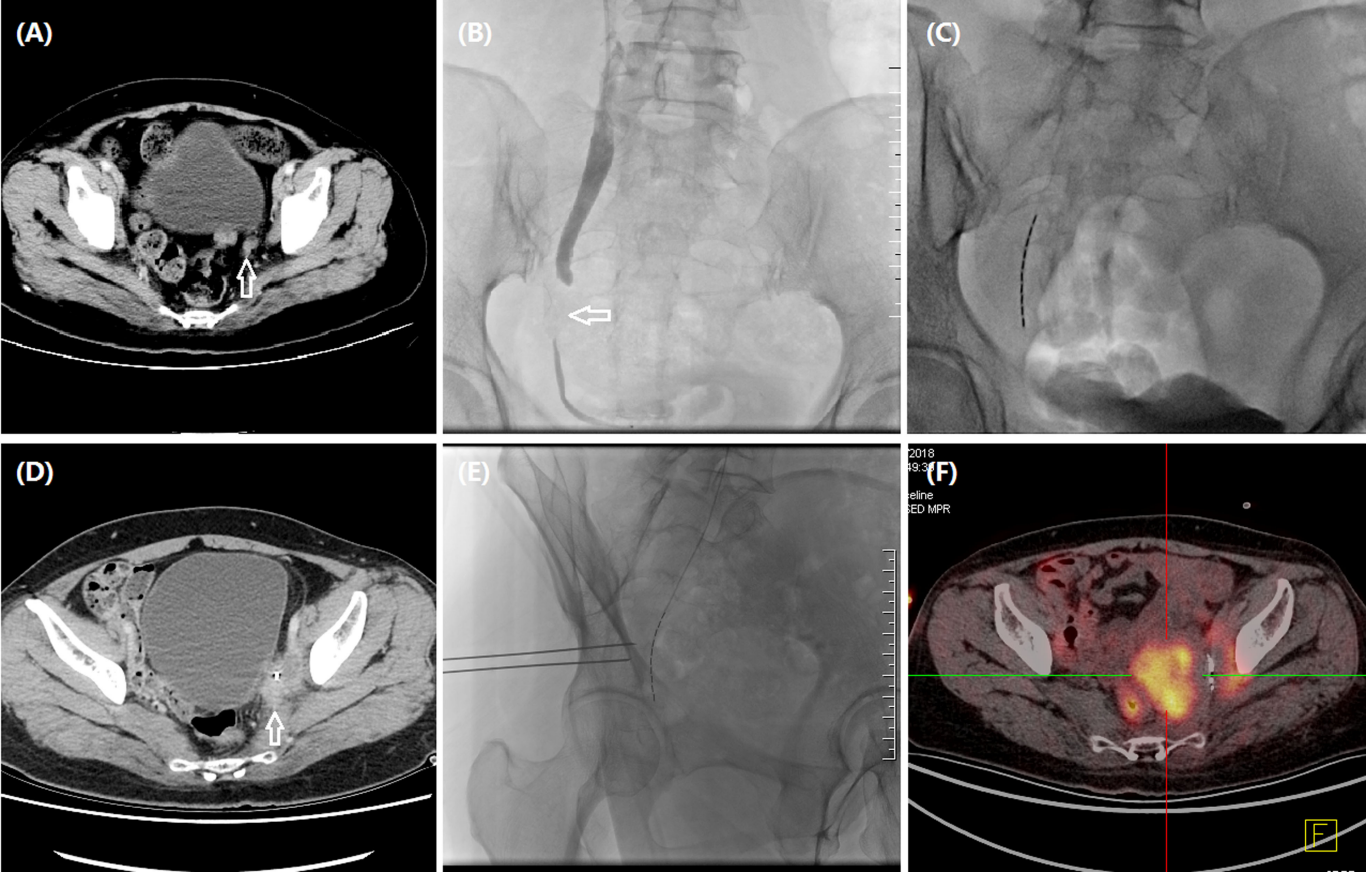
A 48-year woman treated by iodine-125 seed strand for metastatic carcinoma of ureter. (A) CT revealed metastatic carcinoma of left ureter due to recurrent cervical carcinoma (arrow). (B) Urothrography revealed a segmental occlusion (arrow) in the lower segment of left ureteral segment, approximately 27.2 mm in length. (C) Iodine-125 seed strand with 10 seeds was inserted in the occlusive segment. (D) CT revealed an enlarged tumor (arrow) 2.2 months later. (E) Progressive tumors were treated with percutaneous particle implantation, and a total of 10 particles were implanted. (F) Tumor progression was detected by PET-CT after 12.0 months’ follow-up.
